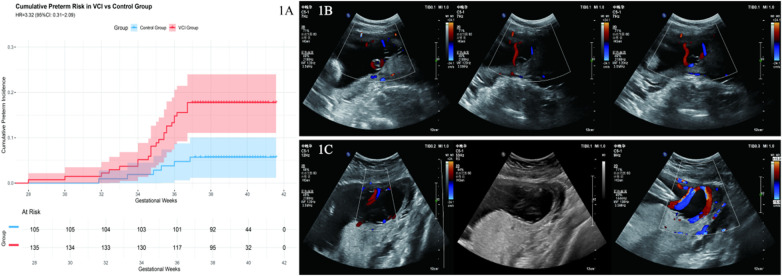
Cumulative risk of preterm birth in VCI and typical ultrasound images. (A) Comparison of cumulative preterm birth risk between the velamentous cord insertion (VCI) group and the control group. Kaplan–Meier curves show that the VCI group (red line) had a significantly higher cumulative risk of preterm birth than the control group (blue line); log-rank *χ*^2^ = 7.77, *P* = 0.005. Hazard ratio = 3.32 (95% CI: 1.36–8.12). Shaded areas represent 95% confidence intervals; numbers at risk at each timepoint is shown below the plot. (B) Ultrasound image of a high-risk VCI case (Patient 1)—a 41-year-old woman at 22 weeks of gestation. Color Doppler shows umbilical vessels (red/blue flow signals) coursing within the fetal membranes without Wharton's jelly protection. Model 4 predicted a 45.4% probability of preterm birth based on advanced maternal age (41 years), minimal growth discordance (BPD_FL_diff = 0.03), and modest Doppler deviation (Z_Doppler = 0.48); delivery occurred at 34 + 4 weeks with a birth weight of 2,020 g. (C) Ultrasound image of a moderate-risk VCI case (Patient 2)—a 39-year-old woman at 23 weeks of gestation. Ultrasound shows umbilical vessels within the membranes with clear flow signals. Model 4 predicted a 28.0% probability of preterm birth based on significant growth discordance (BPD_FL_diff = 1.55) and elevated Doppler deviation (Z_Dopple*r* = 1.29), counterbalanced by younger age relative to high-risk threshold; delivery occurred at 39 + 1 weeks with a birth weight of 2,030 g, indicating fetal growth restriction despite near-term gestational age.
